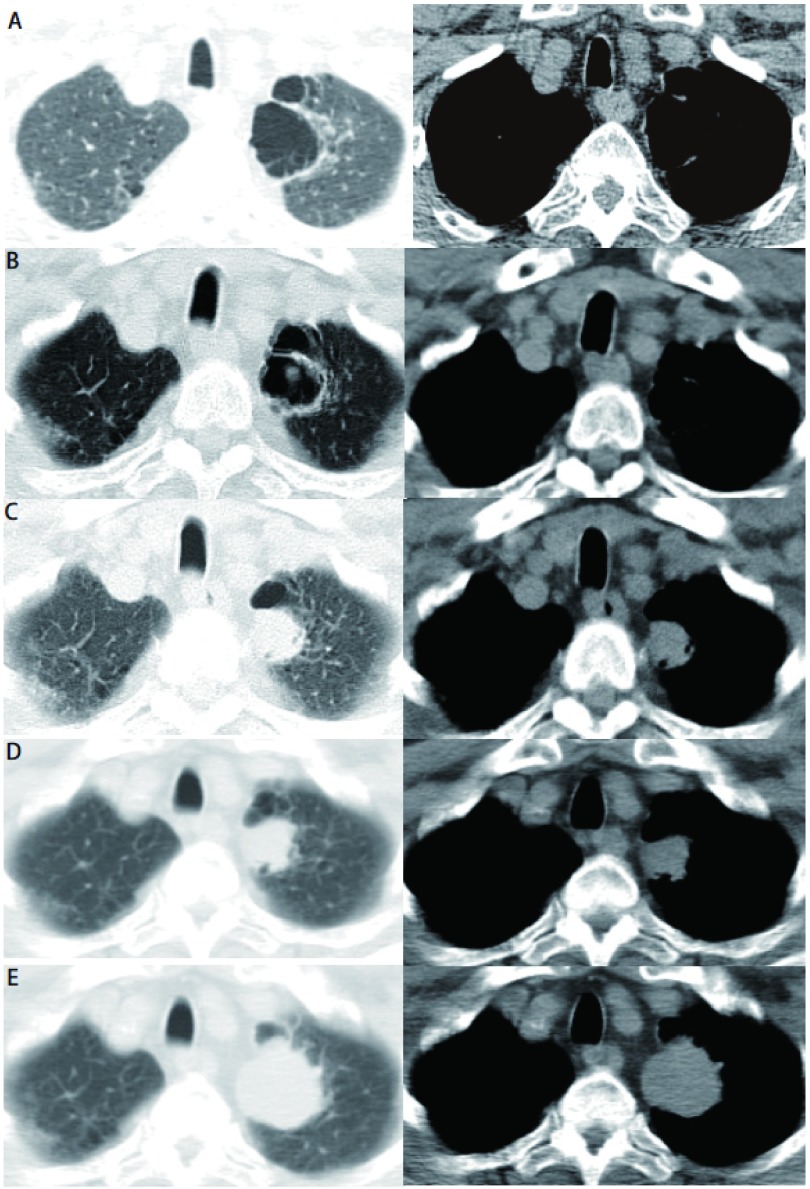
图A-E为病例1病灶由肺大泡到肺部肿块的演变过程。A：CT见左上肺大泡；B：2个月后肺大泡内出现结节；C-E：6个月内结节逐渐增大成为肿块。 Pictures A-E show the lesion experienced a succesion of variations from bullae to mass of case one. A: CT scan shows bullae in the upper lobe of left lung; B: A nodule can be found in the bullae two months later; C-E: The nodule becomes a mass gradually in six months.
